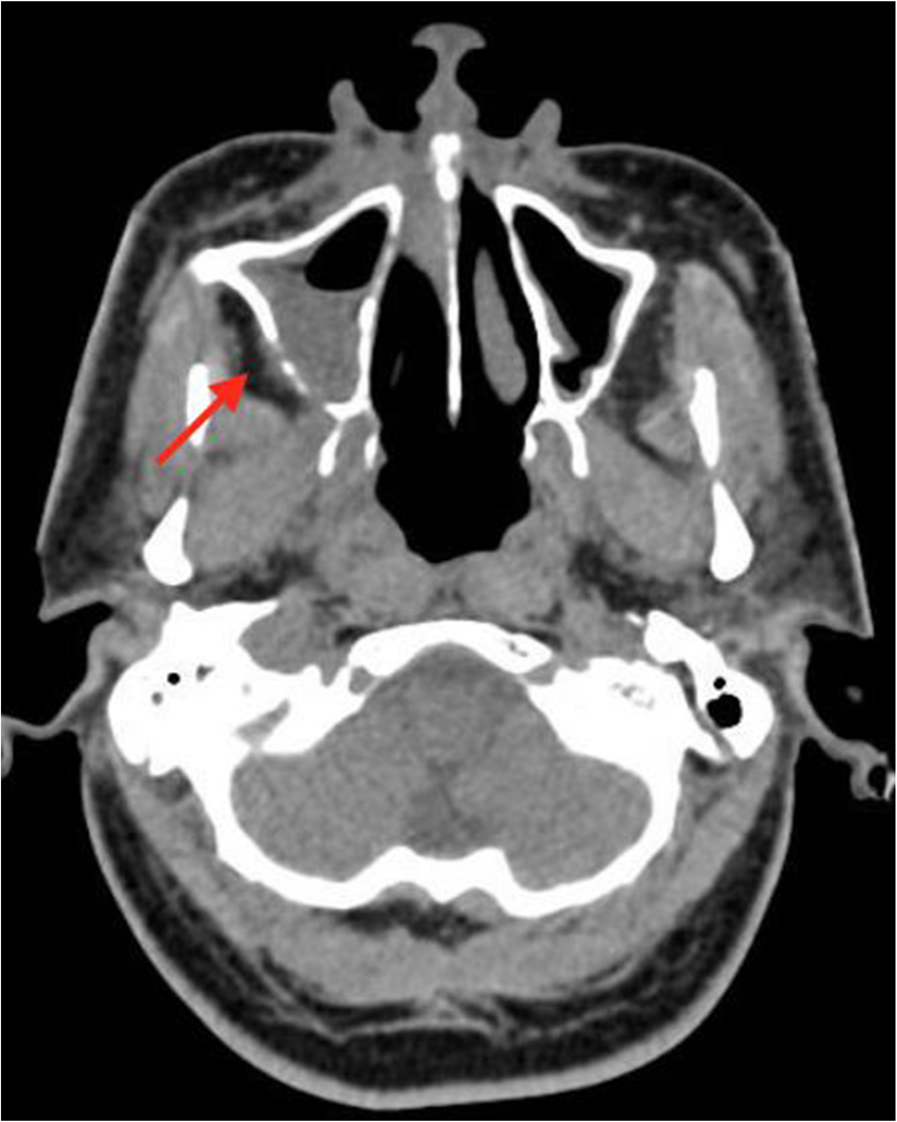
Axial non-contrast CT shows infiltration of the right retroantral fat plane (arrow) indicating invasive disease extending through the posterior wall of the right maxillary sinus
